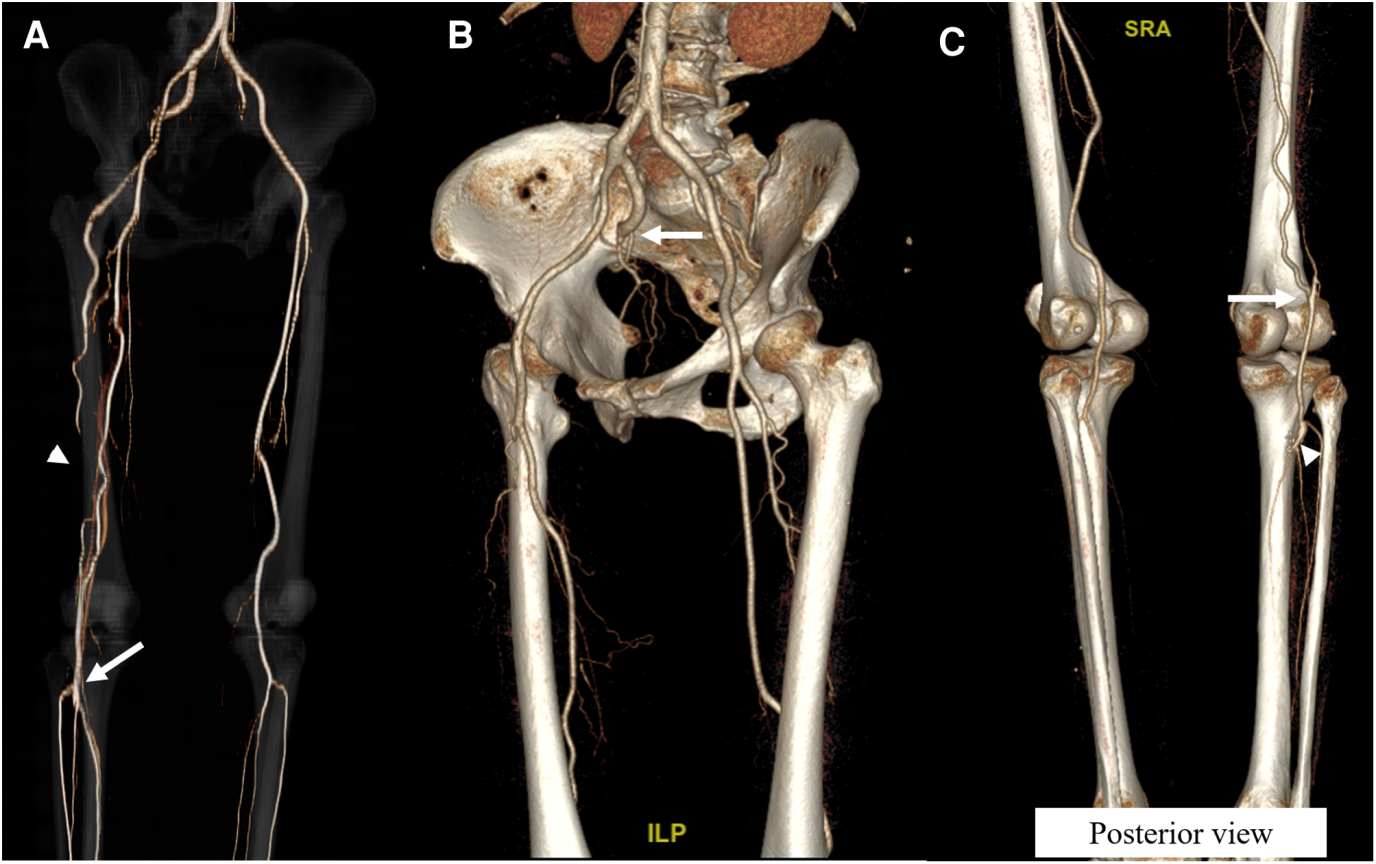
Follow-up computed tomography angiography (CTA) images of the first case. (A) Following the operation, a three-dimensional reconstruction image 1 month after the operation demonstrated a well patent distal popliteal artery (*arrow*) and preserved flow from the collaterals from the deep femoral artery (*arrowhead*). (B) CTA 3 years after the operation revealed the complete occlusion of the persistent sciatic artery from the buttock (*arrow*). (C) Posterior view of the CTA 3 years after the operation demonstrated well patent collaterals from the deep femoral artery (*arrow*) and patent operation site (*arrowhead*).
